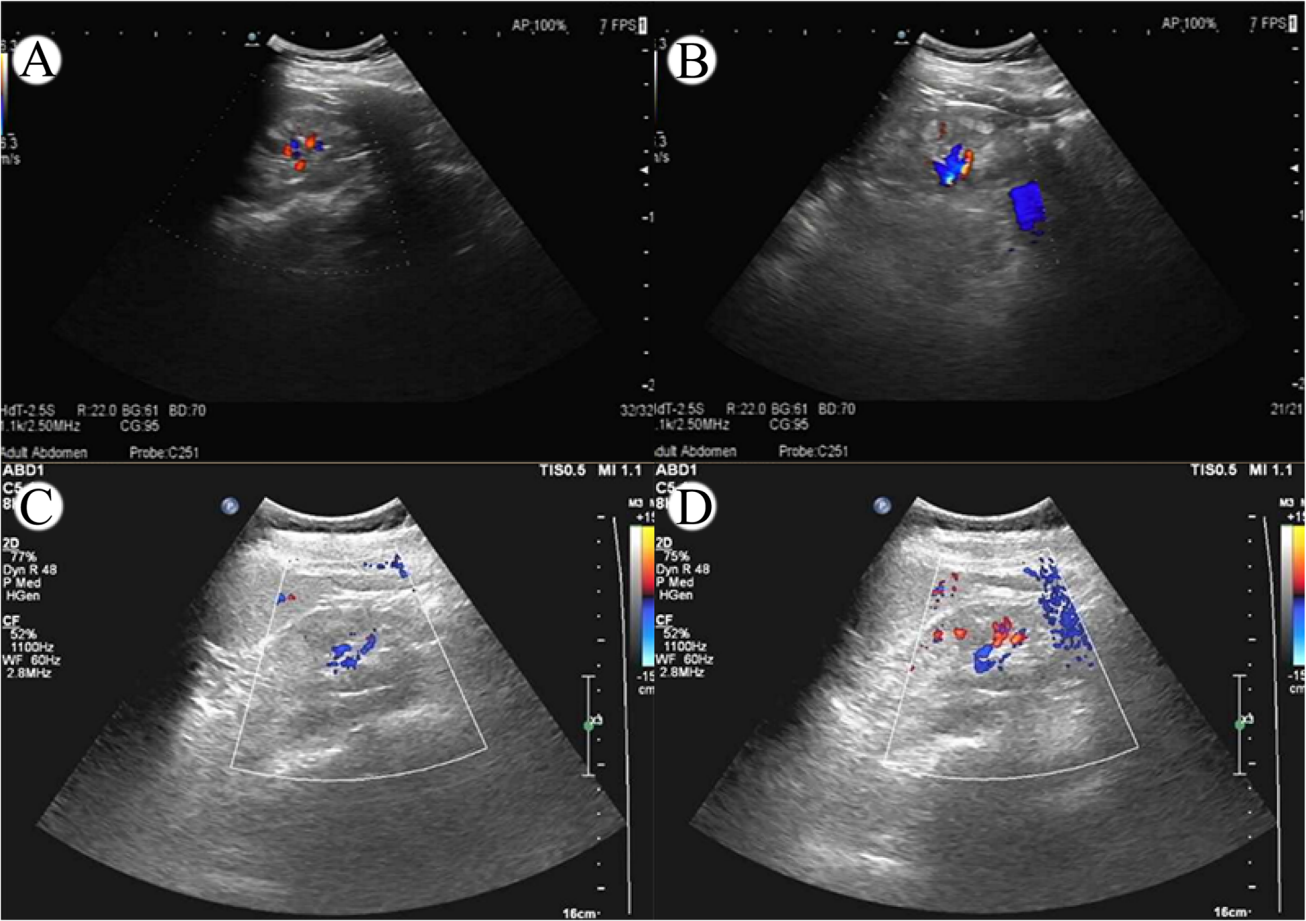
Renal ultrasonography findings in the patient before and after CoQ10 treatment. a, b Increased echogenicity of renal medullae before treatment with CoQ10. c, d Normal kidney echogenicity after treatment with CoQ10
